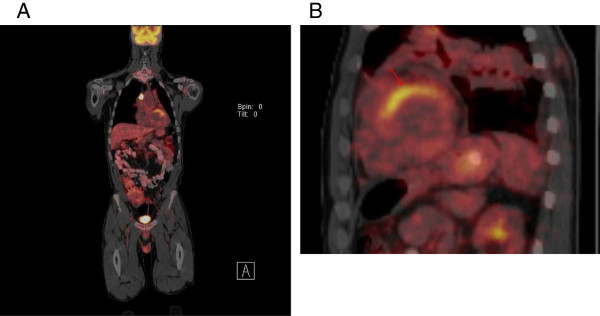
Whole-body FDG PET image in fasting condition shows FDG accumulation in the submandibular region, mediastinal, and right hilum lymph nodes and the heart involving basal and mid anterior wall (A). Short-axis image of fasting FDG-PET of the heart with FDG accumulation in the anterior and anteroseptal wall (B).
